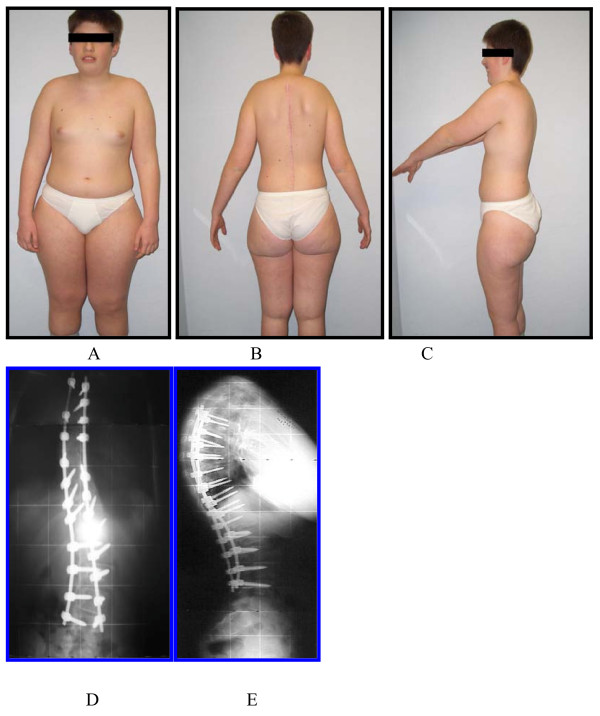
Clinical and radiographic picture at a 2-year follow-up. C.M., clinical and radiographic follow-up in 2007: T4-L4 arthrodesis using only pedicle screws. A notable deformity correction was achieved and is now visible.
